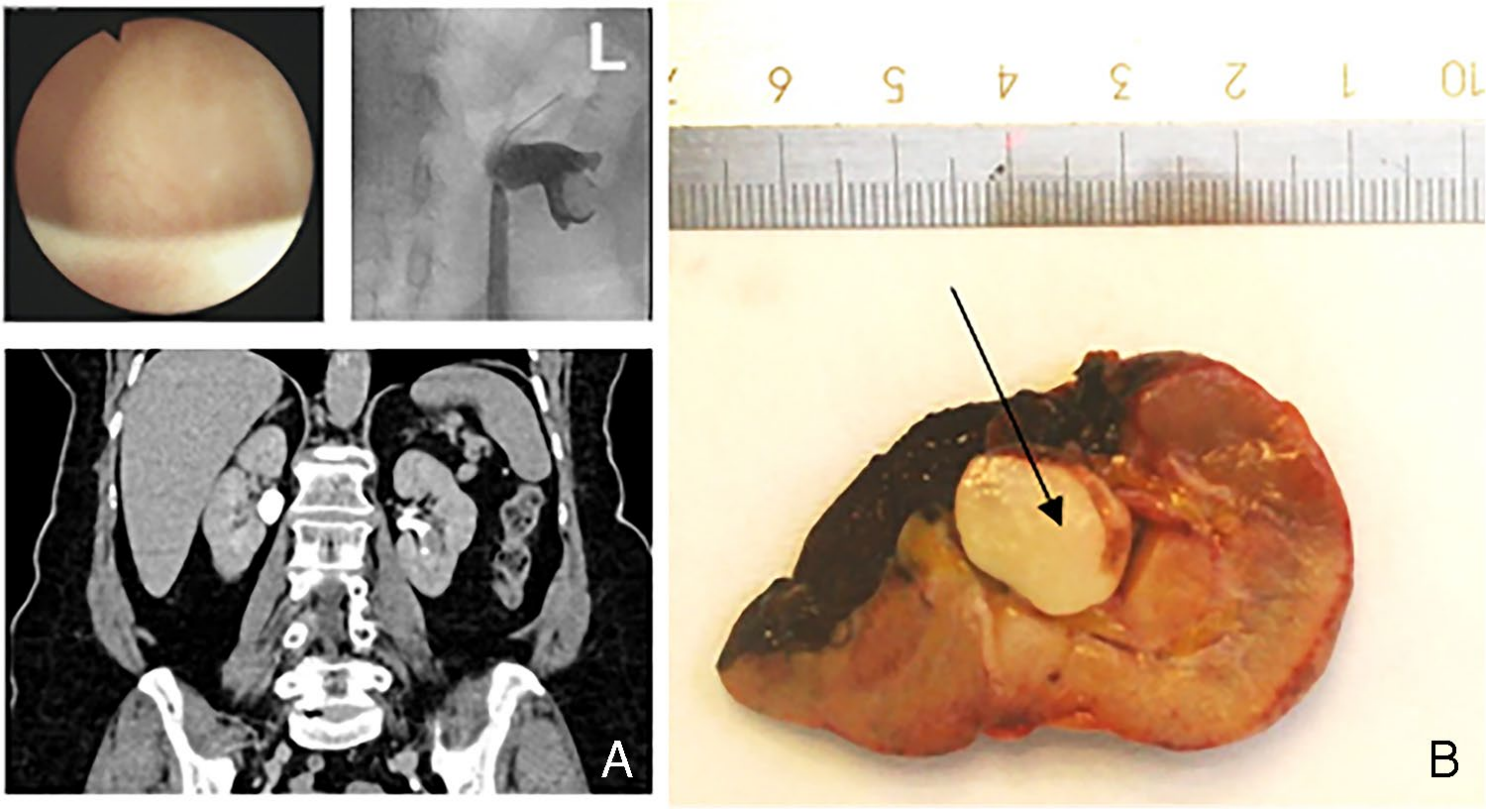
A Computed tomography indicated the presence of a tumour in the lower calyx of the left kidney; meanwhile, during diagnostic ureterorenoscopy (upper left corner), a solid tumour was observed in the lower calyx. B The tumour (black arrow) macroscopically presented as a white and well-circumscribed mass protruding from the renal pelvis
